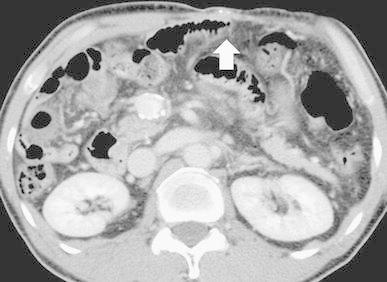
CT scan shows spotty retention of the NBCA mixture in the draining vein in the abdominal wall (*arrow*). There is no recurrence of mesenteric varices (*arrow*)
